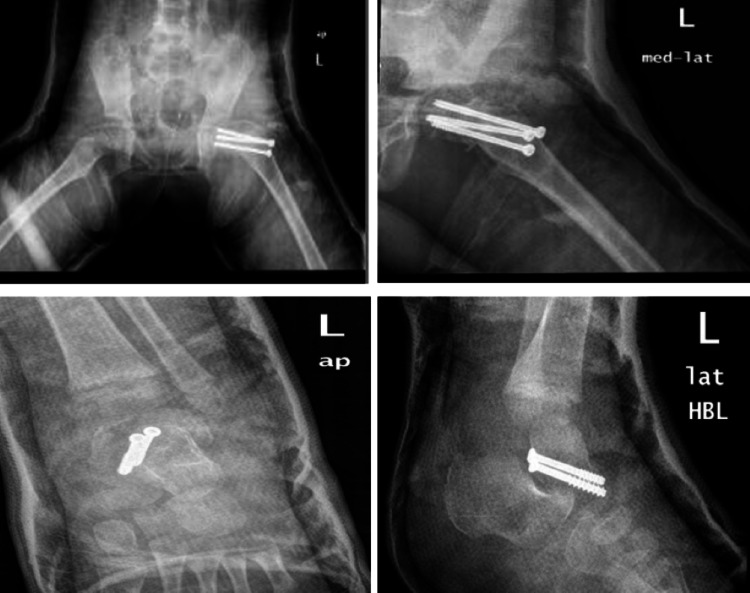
X-ray at two weeks follow-up post-operation
